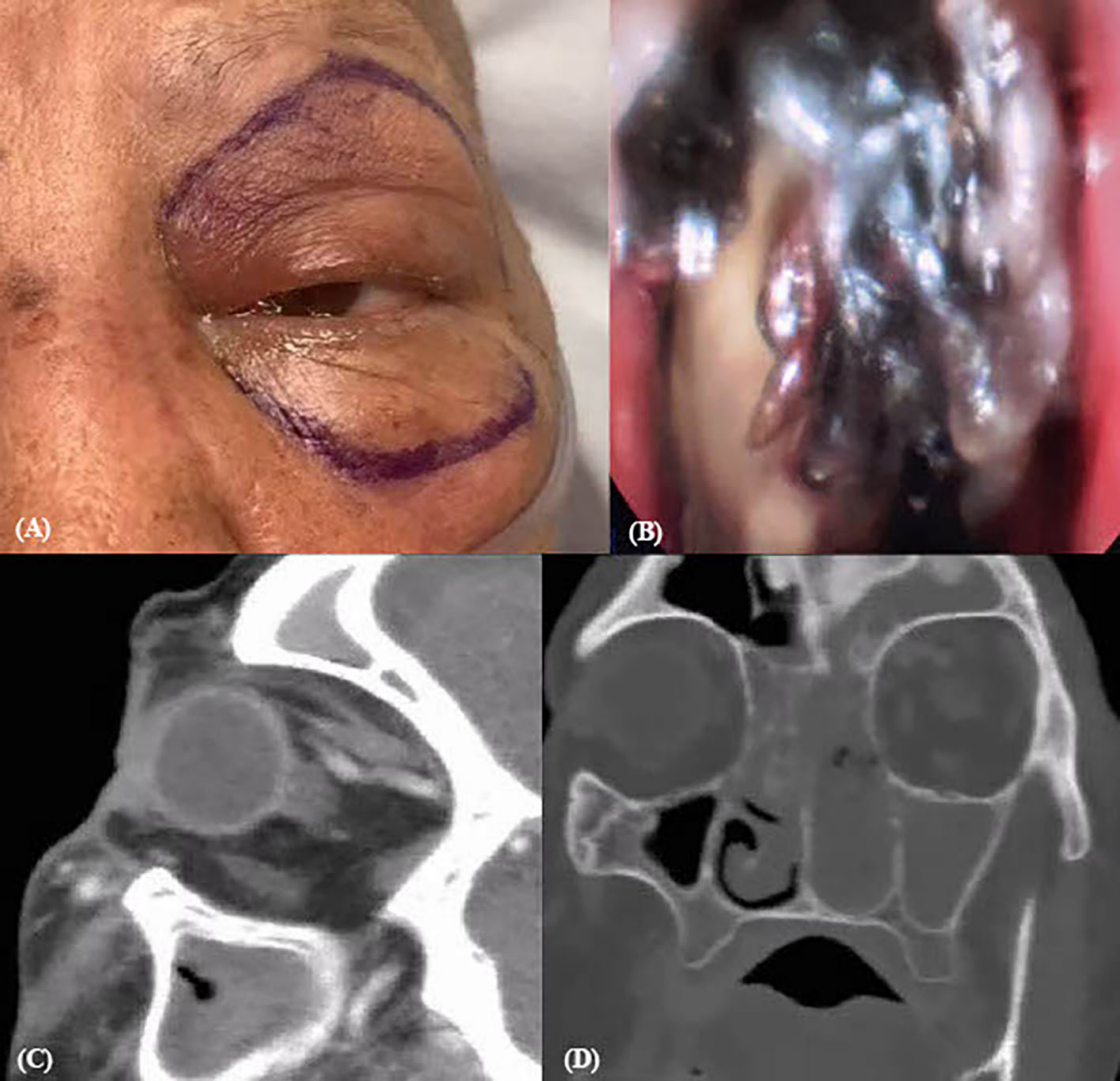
Pre-operative endoscopic and CT contrast imaging of case 2. (A) Pre-operative gross imaging. (B) Fungal growth on ESS. Sagittal (C) and coronal (D) view on CT orbit with contrast showing increased reticulation and stranding around left optic nerve and retro-orbitally.
